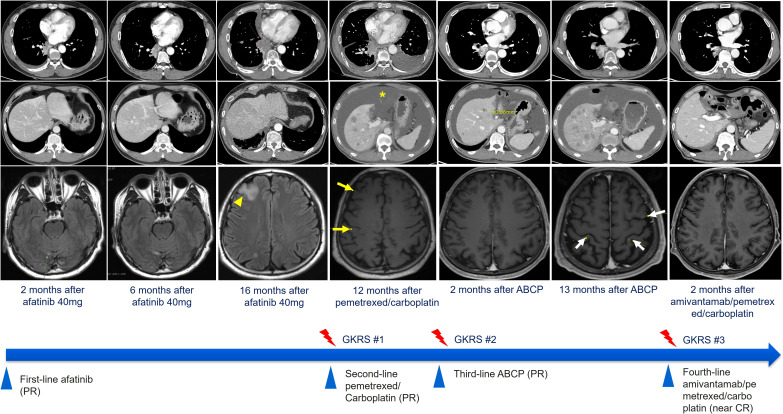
Clinical course with subsequent treatments. The first response evaluation with chest CT and brain MRI showed a marked tumor reduction after first-line afatinib therapy. The response was maintained for 16 months during afatinib treatment. However, pleural metastases progressed, and a new brain metastasis emerged in the right frontal lobe (yellow arrow). Follow-up EGFR testing did not detect the T790M mutation; therefore, the treatment regimen was switched to pemetrexed plus carboplatin, and gamma-knife radiosurgery (GKRS) was performed for the brain lesion. The second-line treatment was effective for 12 months, as evidenced by follow-up chest CT and brain MRI findings. The patient later presented with dyspnea and abdominal pain, and chest and abdominal CT scans revealed markedly aggravated pleural and hepatic metastases with massive ascites, cytologically confirmed as carcinoma peritonei. Brain MRI also showed aggravated brain metastases (arrow). As third-line therapy, a combination of ABCP was initiated along with a second GKRS. This regimen was effective, as demonstrated on CT and brain MRI, and the duration of response lasted 13 months. However, peritoneal seeding and hepatic and brain metastases subsequently recurred, and his performance status deteriorated accordingly. After multidisciplinary counseling with his family, salvage treatment with amivantamab, pemetrexed, and carboplatin was initiated, together with a third GKRS. Remarkably, all metastatic lesions—including those in the liver, peritoneum, and brain—completely resolved in follow-up imaging performed two months later, indicating a complete remission. The response has been sustained for more than nine months, and the patient continues amivantamab and pemetrexed therapy without significant adverse events. ABCP, atezolizumab, bevacizumab, paclitaxel, and carboplatin; PR, partial response; CR, complete response.
